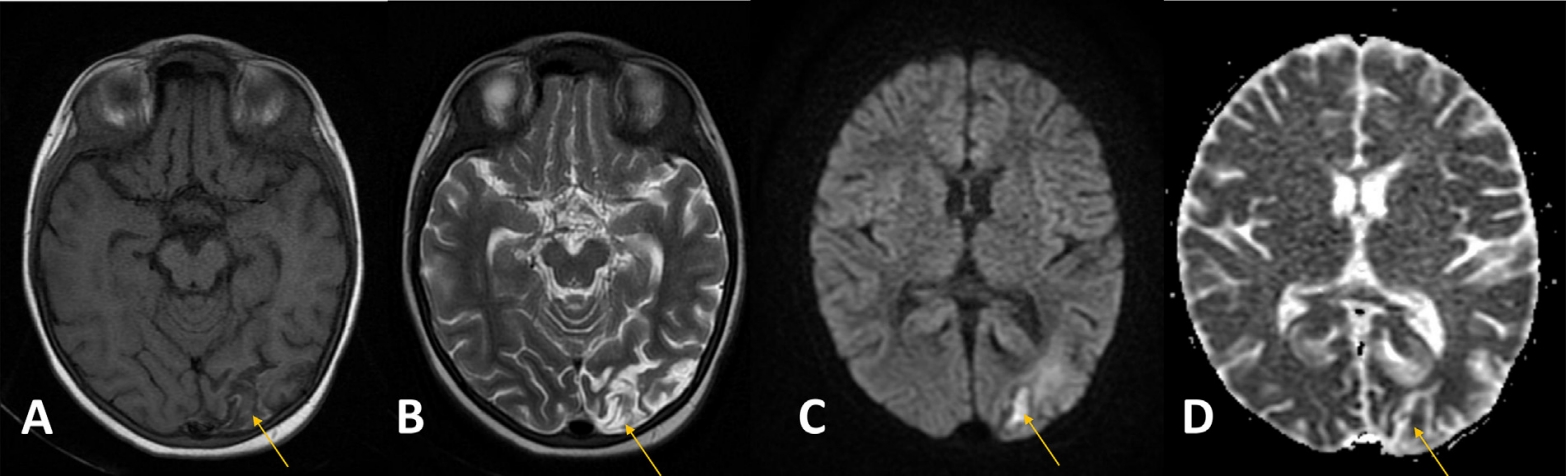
T1 (A), T2 (B), DWI (C) and ADC (D), T1 Iso-hypointense, T2 iso-hyperintense area in the left occipital cortex (arrow) demonstrating diffusion restriction corresponding to ADC mapping representing acute infarct. T1 GYRAL hyperintensity is also seen in the left occipital lobe, representing laminar necrosis secondary to a prior event of ischemia (arrowhead).
